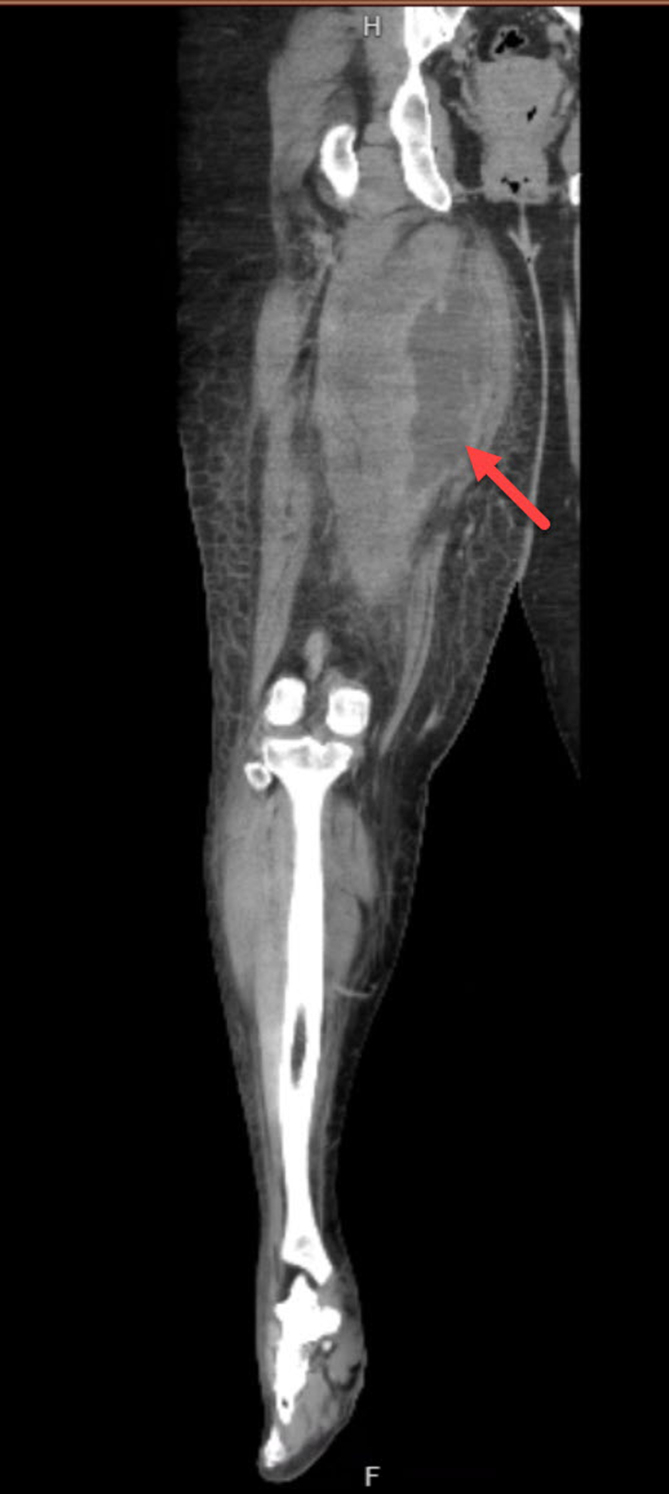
Computed tomography of right leg showing a 19 × 7 × 6.2 cm fluid collection (red arrow).
